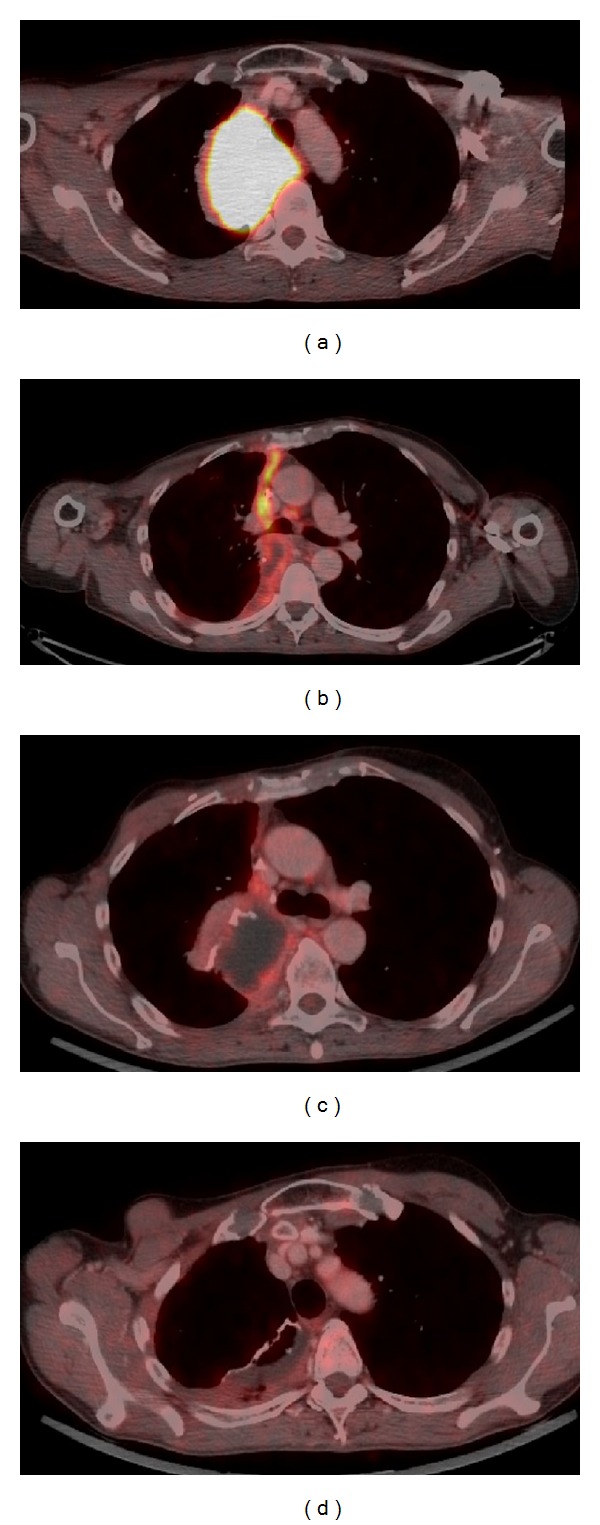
18-FDG PET-CT scan with high FDG uptake in a right paramediastinal mass (a). Postoperative scans showing residual FDG uptake one month after surgery (b), a decrease in intensity after immunomodulation with intravenous immunoglobulins 4 months later (c), and sustained response at one-year followup (d).
